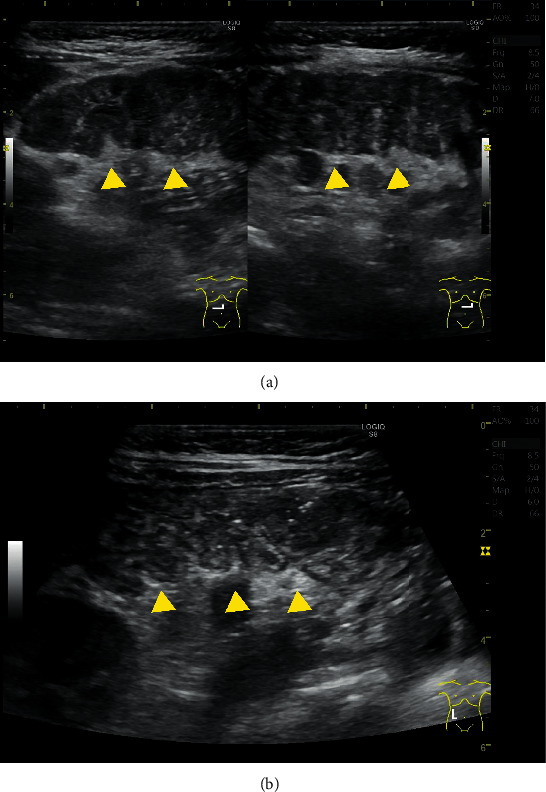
Abdominal ultrasonography revealing intraperitoneal lymphadenopathy, wall thickness of the entire colon.
